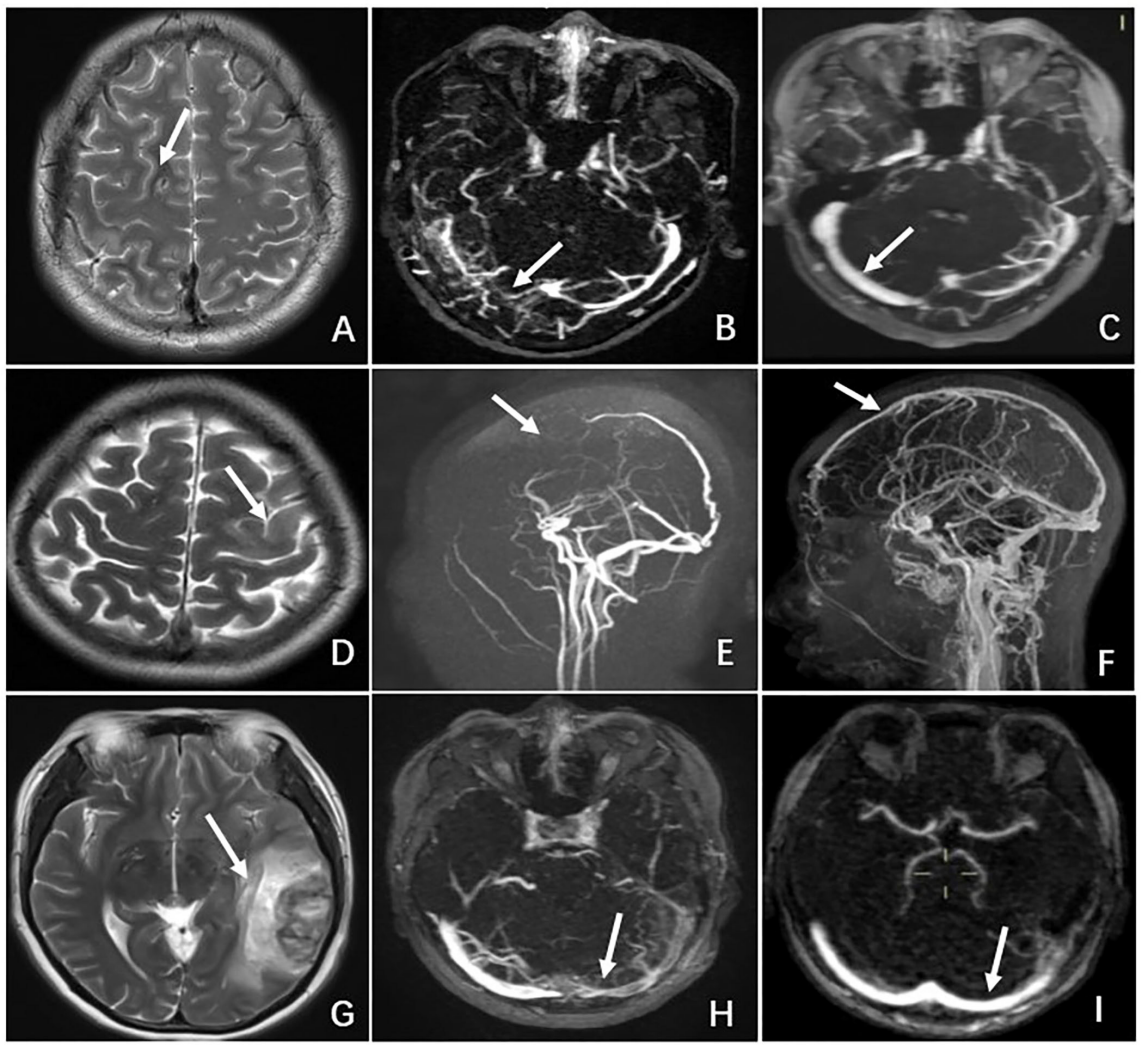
Comparison of radiographic features of CVT between admission and discharge follow-up in 3 cases. Patient N2: (A) MRI on admission showing a hyperintense lesion in the right frontal lobe on T2-weighted MRI. (B) MRV on admission revealing occlusion of the right transverse sinus. (C) MRV on 6-month follow-up demonstrating recanalization of the right transverse sinus. Patient N3. (D) MRI on admission showing a hyperintense lesion in the left frontal lobe on T2-weighted MRI. (E) MRV on admission revealing occlusion of the superior sagittal sinus and cortical vein. (F) MRV on 12-month follow-up demonstrating recanalization of the superior sagittal sinus and cortical vein. Patient N5. (G) MRI on admission showing a hyper-and hypo-density lesion in the left temporal lobe on T2-weighted MRI. (H) MRV on admission revealing occlusion of the left transverse sinus. (I) MRV on 6-month follow-up demonstrating recanalization of the left transverse sinus.
